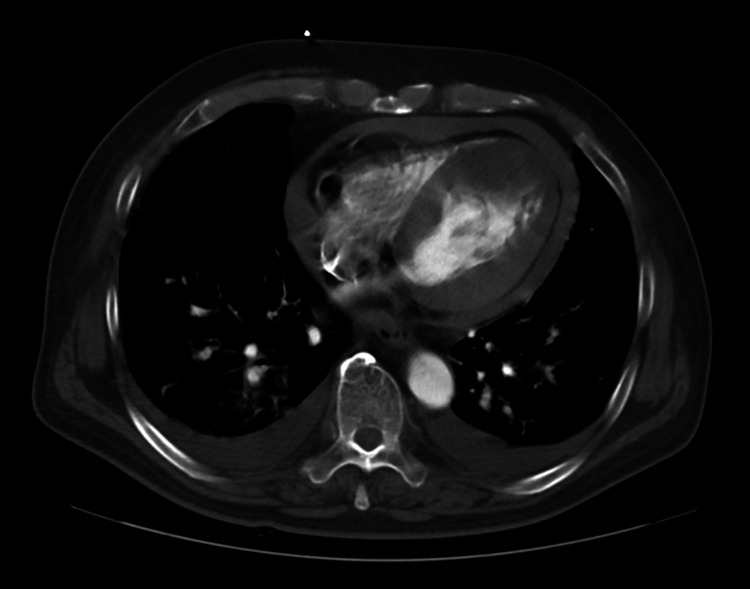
Computed tomography showing a circumferential hemopericardium
